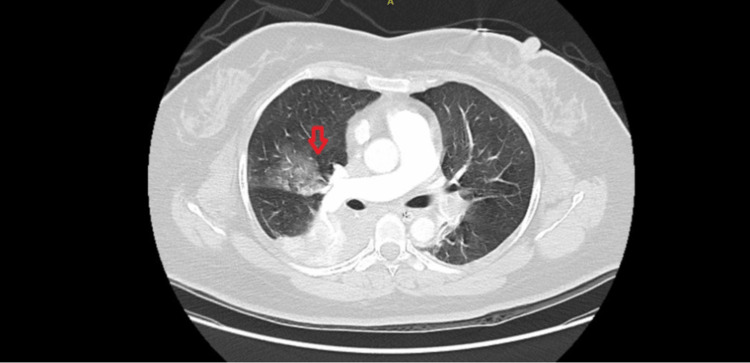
CT-pulmonary angiography: sagittal view showing consolidation in the lateral segment of the right middle lobe with volume loss suggestive of infarcts
